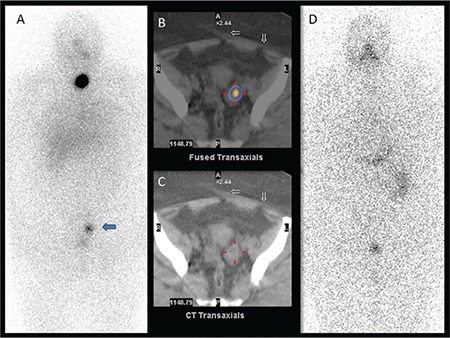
On anterior image of post-therapeutic I-131 whole body scan (A), in addition to residual thyroid tissue, there was a focal I-131 accumulation over the left side of the bladder (arrow). Fused single photon emission computed tomography/computed tomography (CT) image (B) showed that the I-131 uptake was localized to the left ovary and a hypodense cystic area in the left ovary was identified on CT image (C). The followup diagnostic I-131 scan (D) was normal and the thyroglobulin level was undetectable (<0.2 ng/mL)
